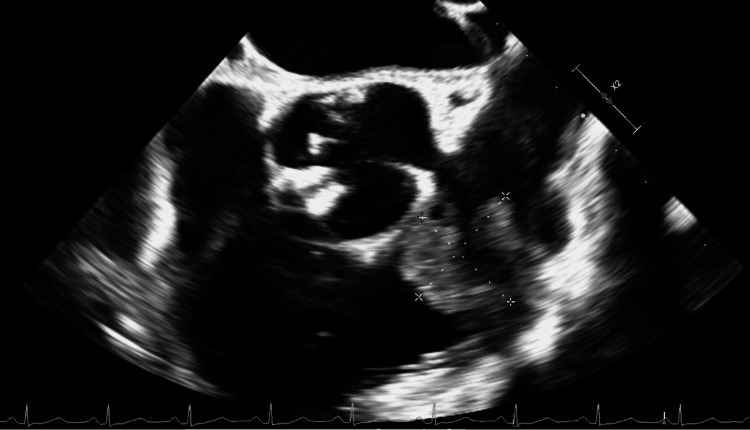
Transesophageal echocardiogram confirming the presence of the mass on the mid-esophageal aortic valve short axis view.
